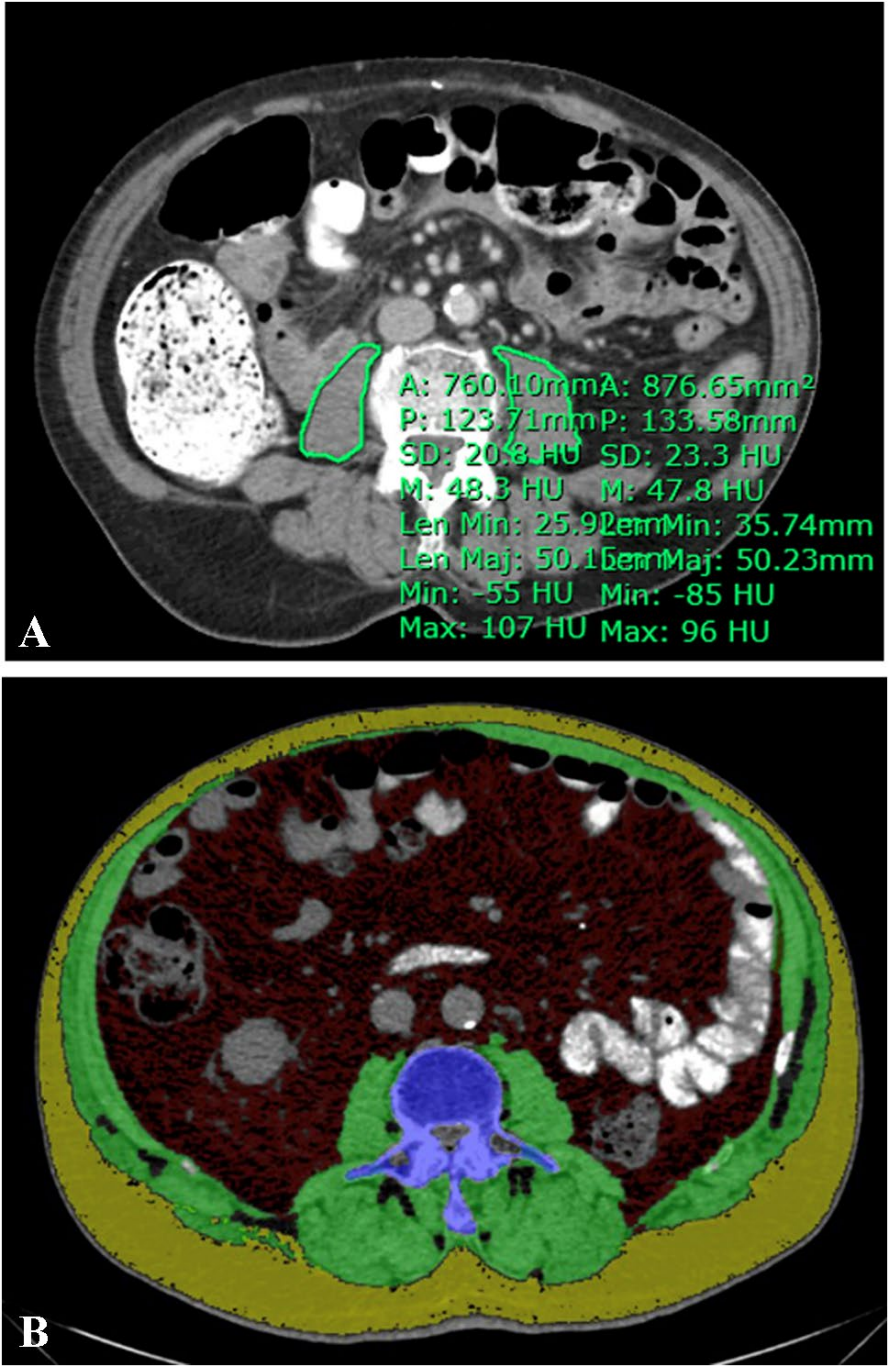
CT image showing the segmentation of psoas muscles (A), visceral fat area (B-red), and subcutaneous fat are (B-yellow)
